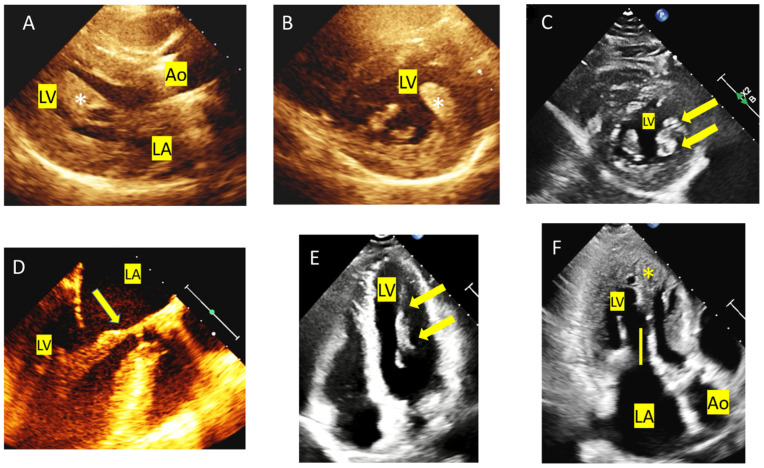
Anomalies of the mitral valve (MV) apparatus. (A) Parasternal long axis view showing an anteriorly displaced and hypertrophied papillary muscle (asterisk). (B,C) Parasternal short axes of the LV: a hypertrophic anterolateral papillary muscle (B, asterisk) and a bifid one (C, arrows). (D) TEE showing the insertion of a head of the anterolateral papillary muscle directly into the mid portion of the anterior mitral valve leaflet (arrow). (E) Four-chamber apical view showing an abnormal apical insertion of the papillary muscle that is also anteriorly displaced (arrows). (F) Three-chamber apical view that allows the measurement of a long anterior mitral leaflet (yellow bar); asterisk showing the anterolateral papillary muscle.
